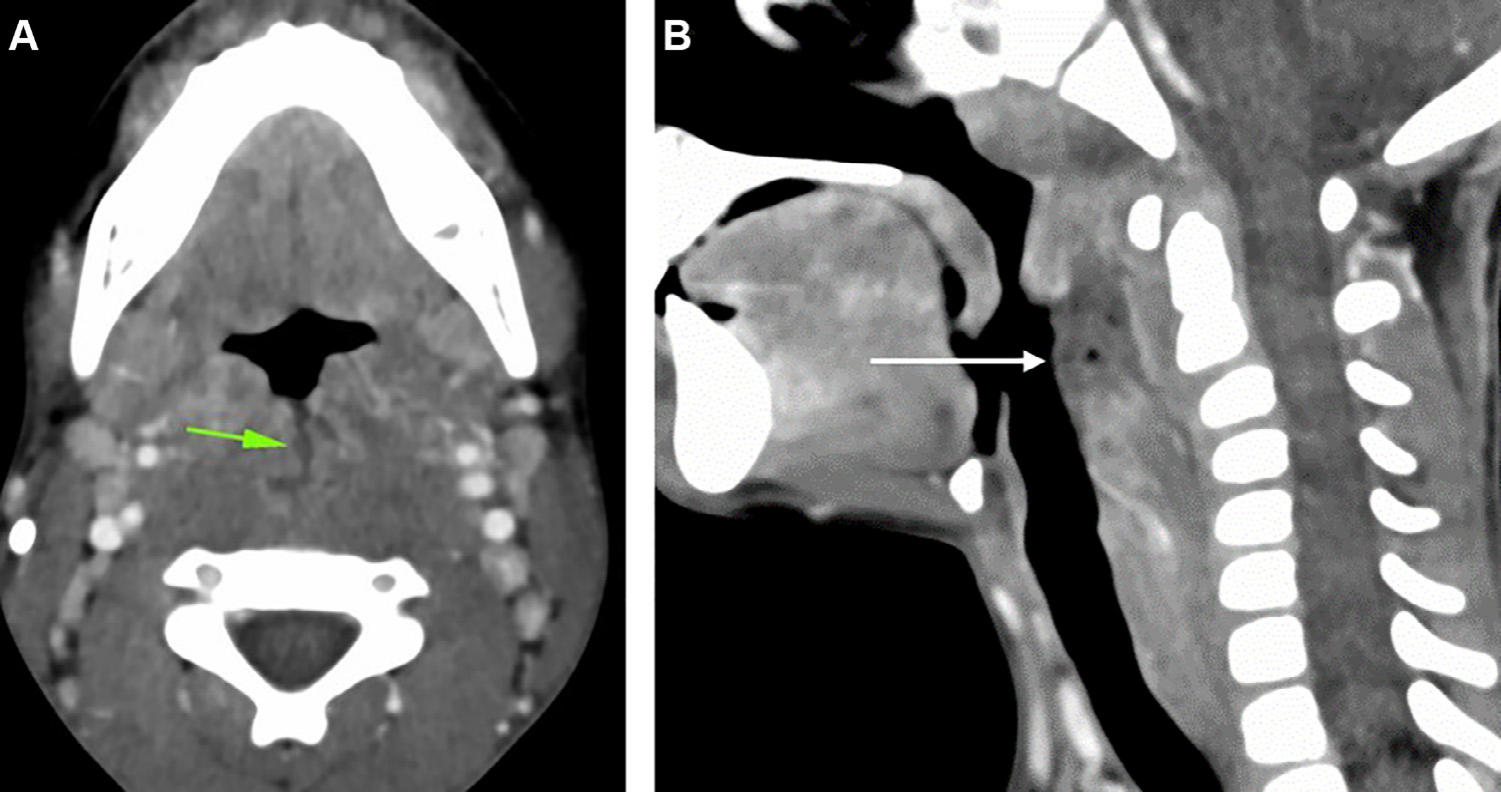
CT showing a median breach of the oropharyngeal mucosa, contiguous with a collection of retropharyngeal fluid containing air bubbles (A and B).
